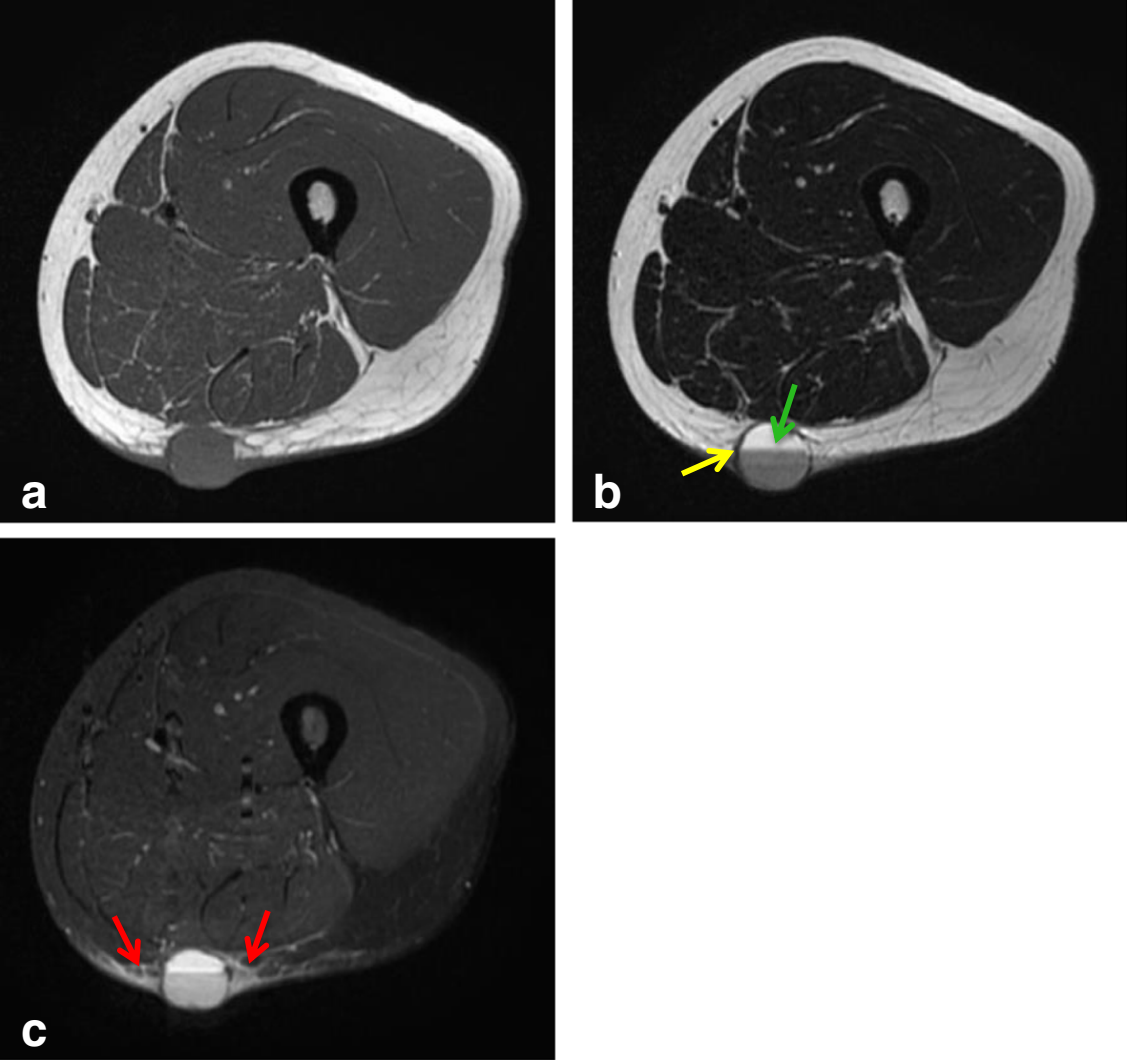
A 28-year-old woman diagnosed with angiomatoid fibrous histiocytoma (case 1): (a) T1-weighted spin echo, (b) T2-weighted spin echo, and (c) STIR images. A 21 × 23 × 22-mm well-circumscribed, round mass is present in the subcutaneous fat of the posterior right thigh. The lesion is homogeneously hypointense on T1 WI and presents fluid–fluid level (*green arrow*) and pseudocapsule (*yellow arrow*) on T2 WI. STIR shows peritumoral edema (*red arrows*)
